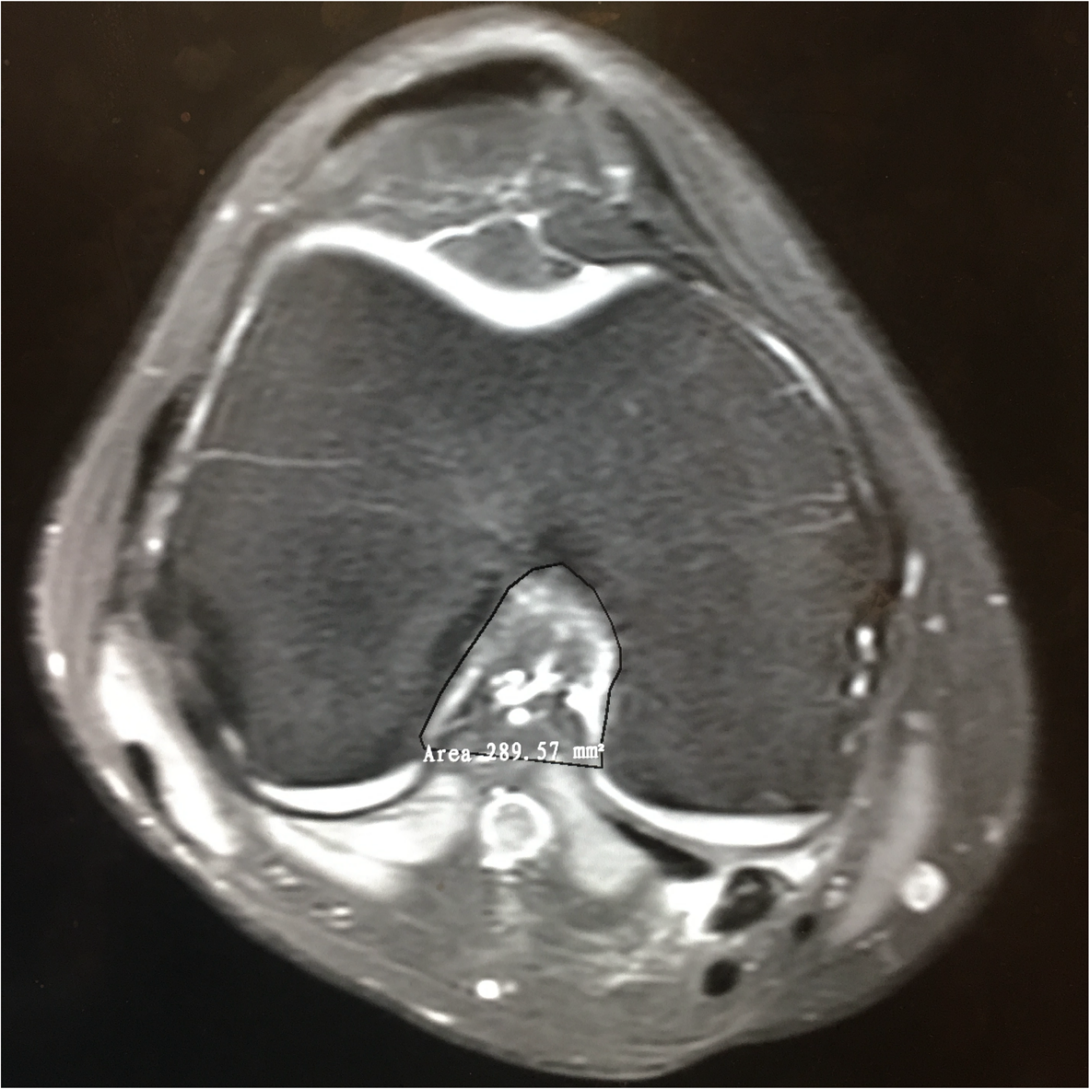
The axial slice of the knee MRI shows the measurement of femoral intercondylar notch volume. The black curve traces the boundaries of the femoral notch. And the number beside the tracing place is the area calculated by the software itself
